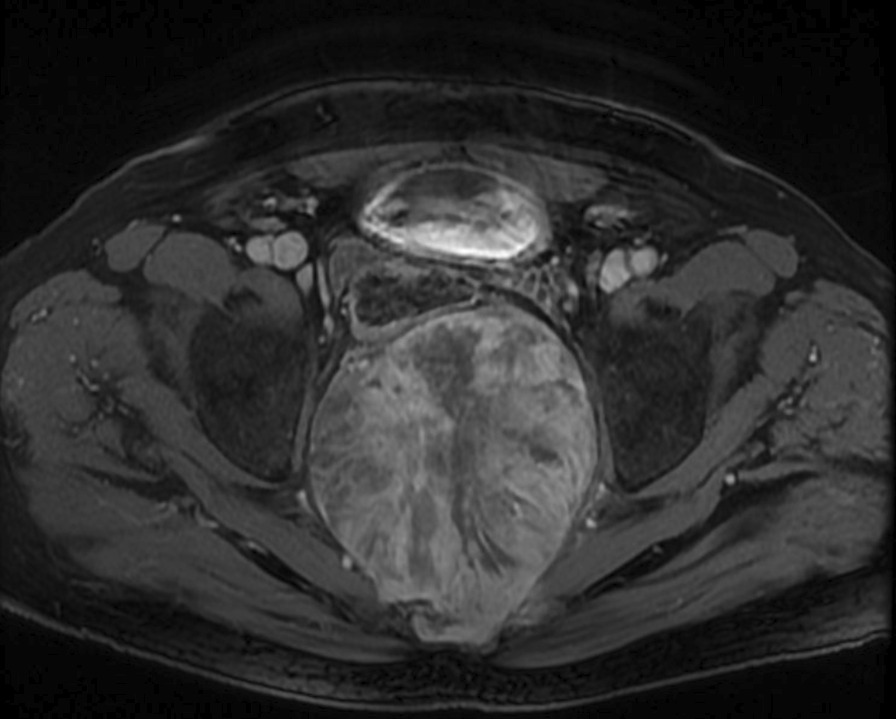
Magnetic resonance imaging of the pelvis showed a large midline, well-defined, oval shaped lesion replacing the sacrococcygeal portion of the spine, with extension to the presacral region
